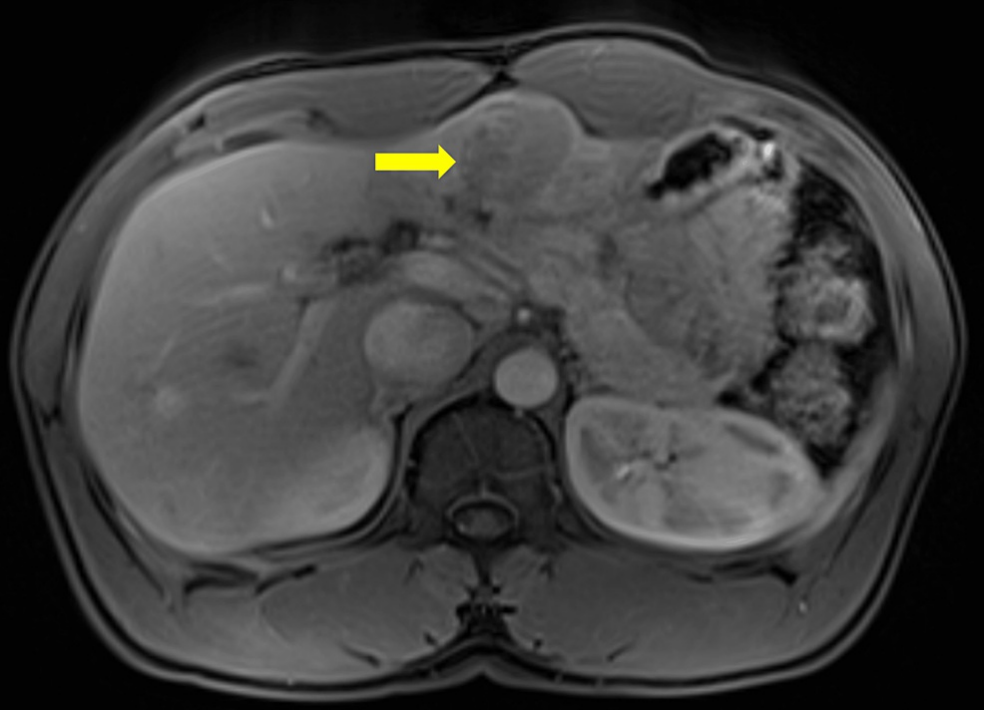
T1-weight axial plane post-contrast venous phase MRI abdomen MRI: Magnetic Resonance Imaging Lesion was measured 4.5 cm and localized to left lateral segments 2 and 3, mildly hypointense with diffuse mild and heterogeneous arterial phase hyperenhancement along with washout and capsule formation.
